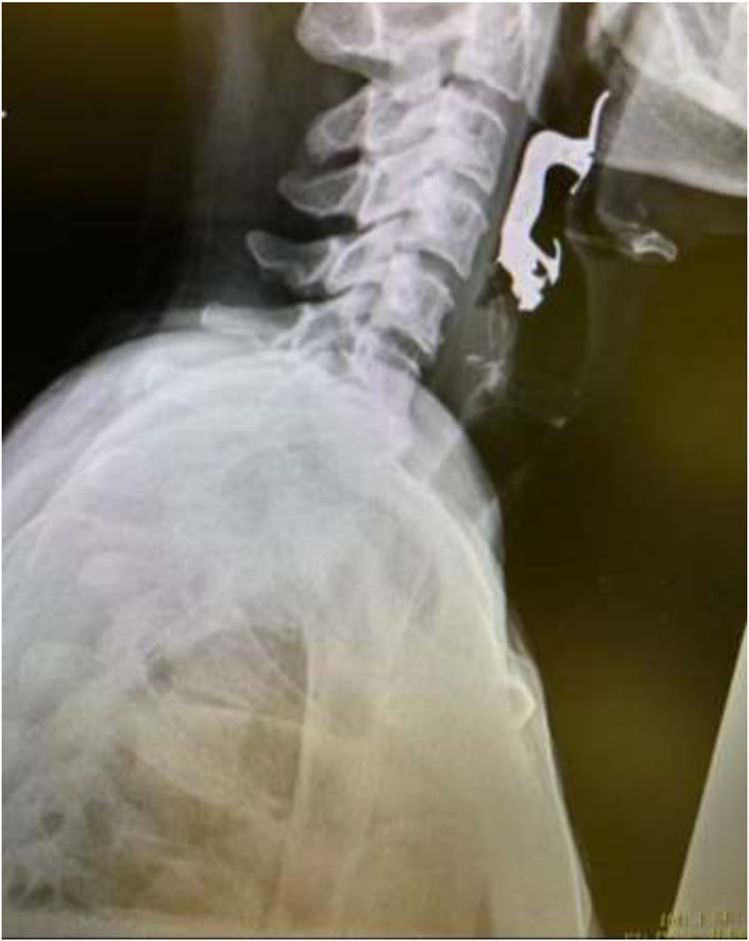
Lateral neck X-ray showing radio-opaque object in the upper airway.
